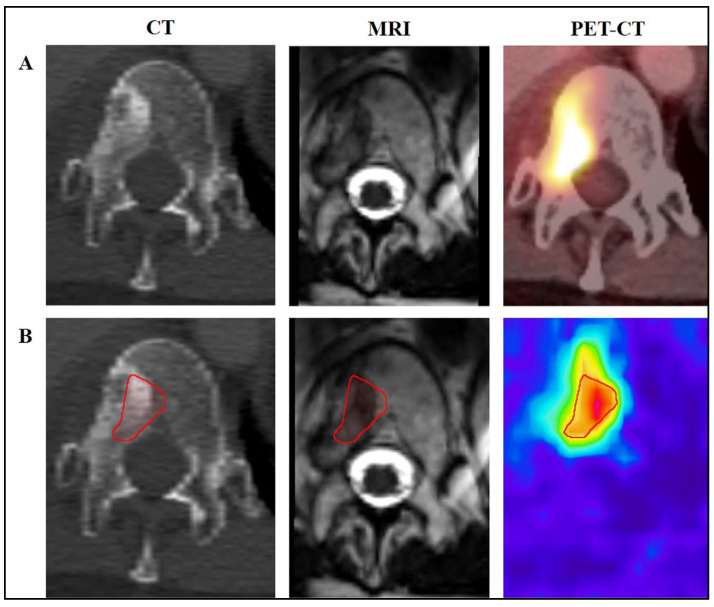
A 54-year-old patient with a recurrence of dedifferentiated (G3) chondrosarcoma at the level of the thoracic spine (Th 12). (A) Preoperative imaging as part of the staging including CT, MRI, and PET-CT. (B) Preoperative fusion of CT imaging, MR imaging, and PET-CT and marking of the tumor in its total size or, as in this example, marking of the region with the highest activity in PET-CT using Smartbrush software.
